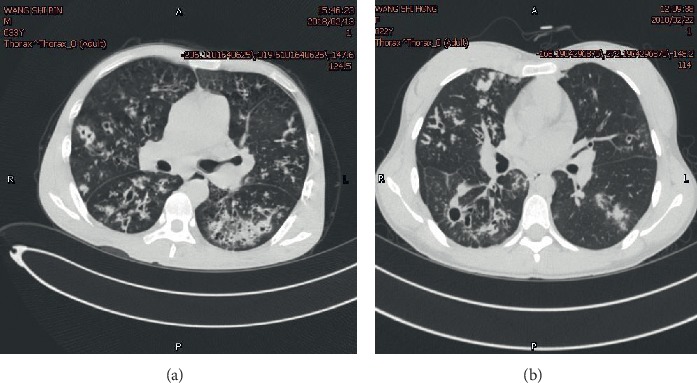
A is the image of chest CT from the male and B is from the female. Both of the images show sacculated bronchiectasis and patchy opacity in bilateral lung field. (a) The brother's chest CT. (b) The sister's chest CT.
